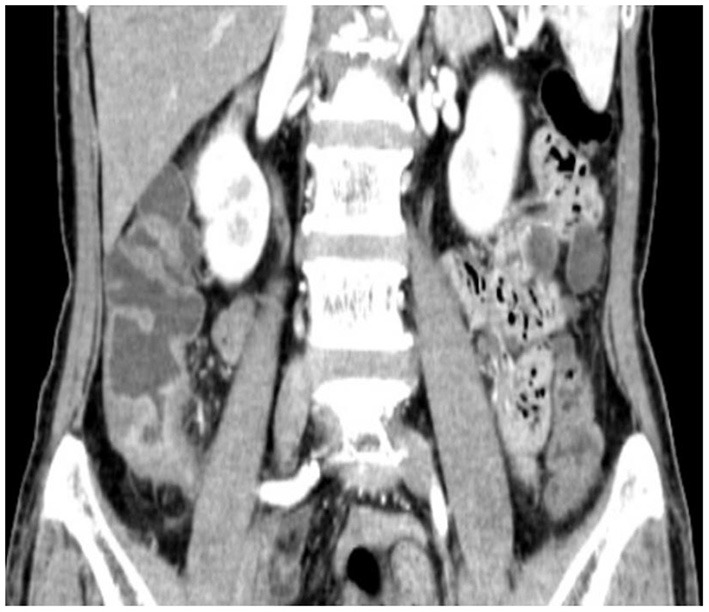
CT showed that the ileocecal intestinal wall was thickened.
